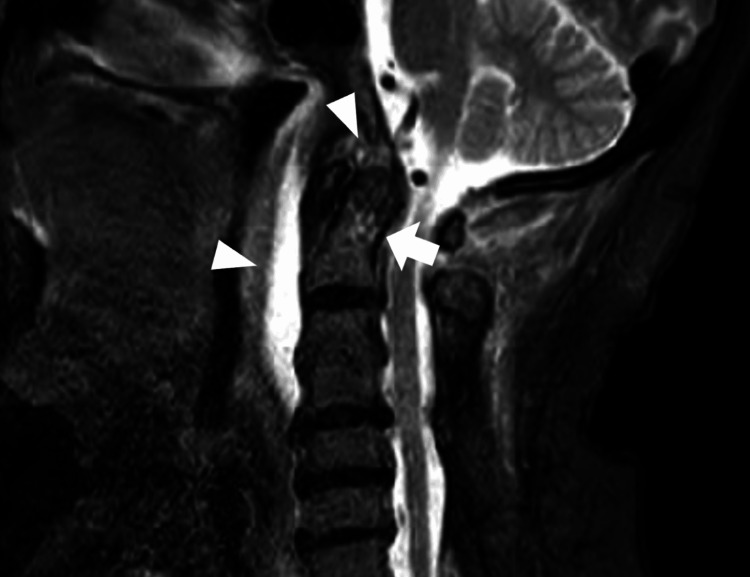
Cervical spine MRI (STIR) showing high signal intensity in the odontoid process (arrow) and fluid accumulation around the odontoid process and anterior to C1-C3 (arrowhead). STIR: Short Tau Inversion Recovery.
